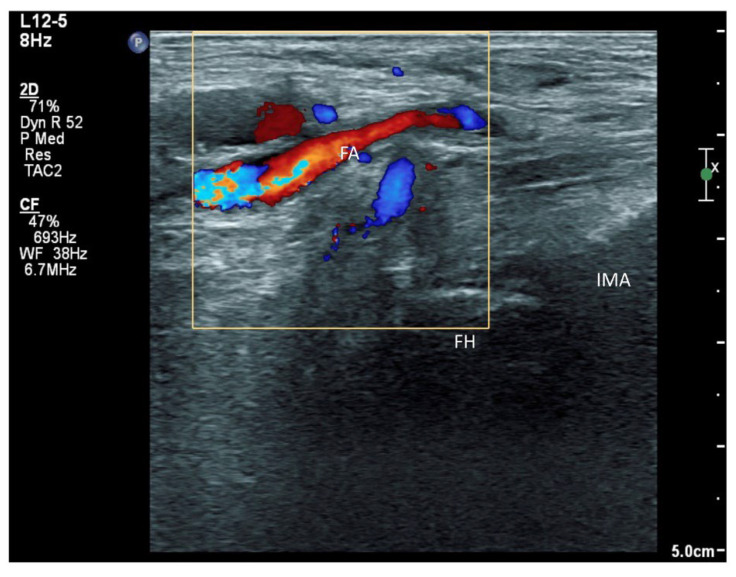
Doppler signal of the femoral artery and its branches when scanning the inferomedial acetabulum by the classical oblique approach. FH: femoral head, FA: femoral artery and branches, IMA: inferomedial acetabulum.
